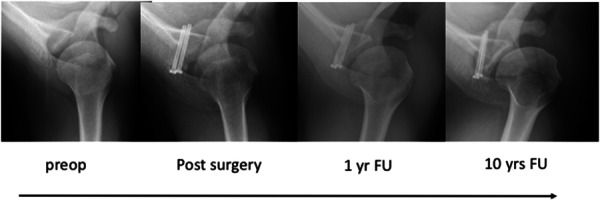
Standard axial radiography over time in a patient without screw removal. FU, follow‐up.
